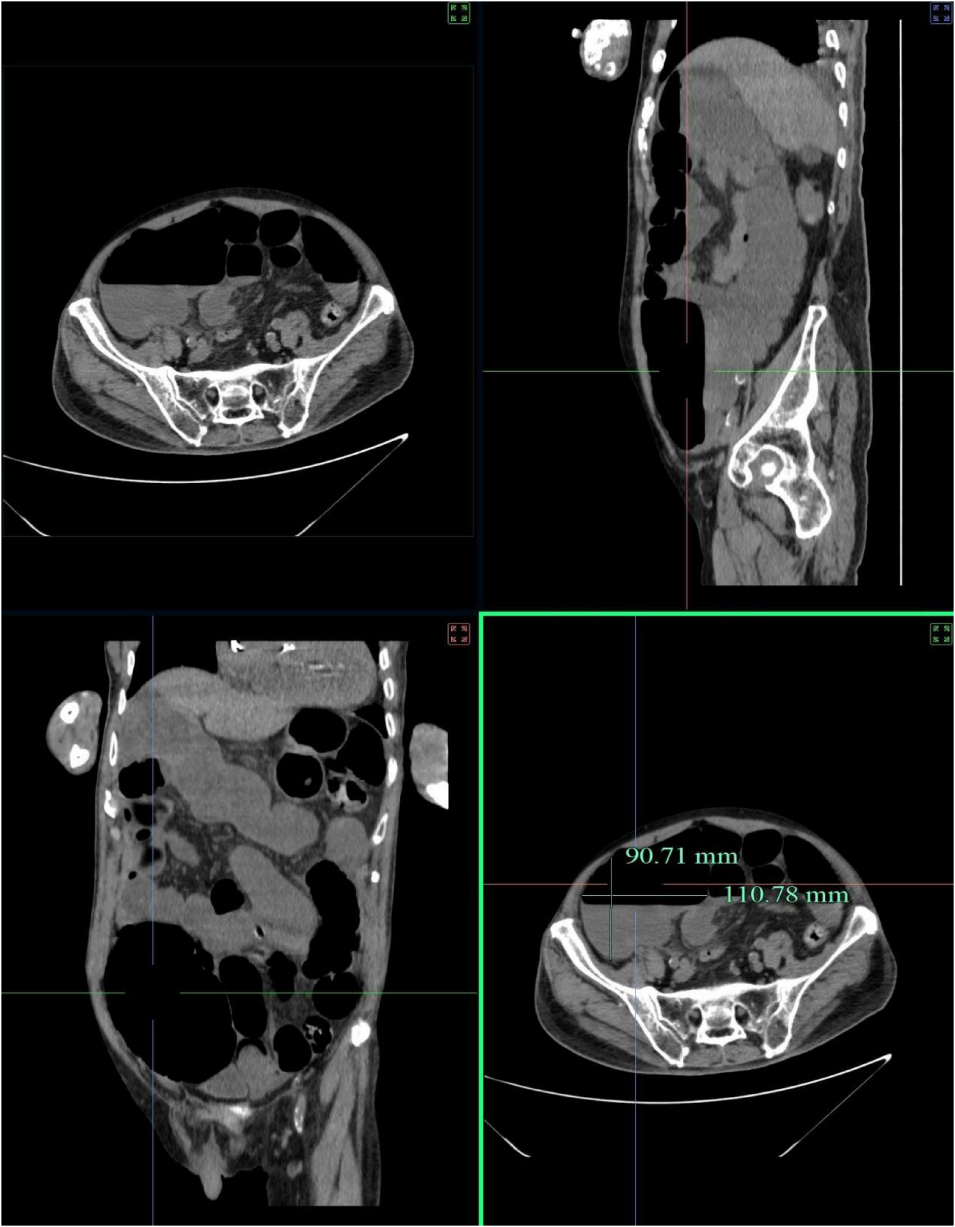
Admission sagittal CT of the abdomen demonstrating intestinal obstruction, characterized by multiple air-fluid levels within dilated bowel loops and marked abdominal distension. The widest part of the horizontal plane was 90.71 mm × 110.78 mm.
